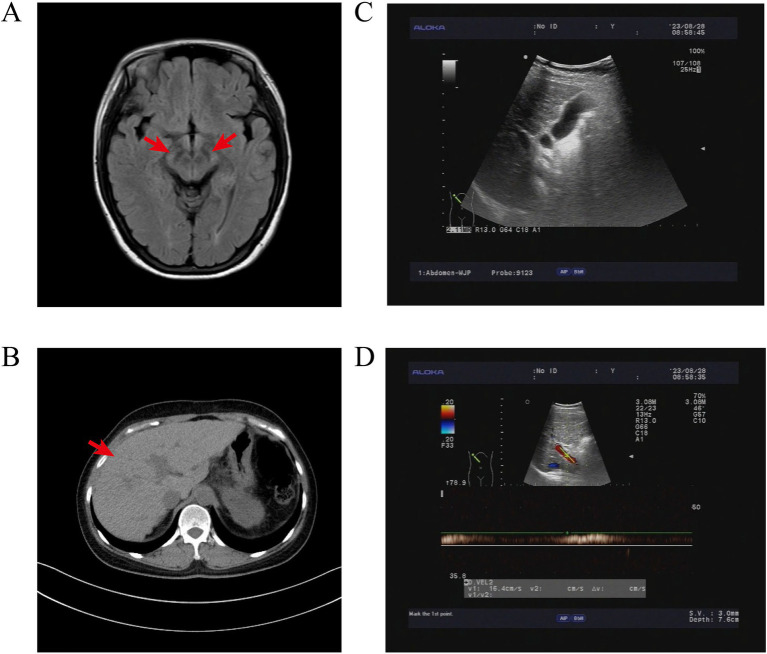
Imaging findings of the patient with WD. (A) Cranial MRI T1WI showed symmetrical abnormal signals in the bilateral cerebral peduncles, consistent with the typical manifestation of central nervous system involvement in WD; (B) chest CT revealed an irregular liver contour, uneven margin, and heterogeneous hepatic parenchymal density; no splenic structure was observed in the splenic region, suggesting post-splenectomy status; (C) liver color Doppler ultrasound demonstrated coarse and enhanced intrahepatic echoes with uneven distribution, suggesting cirrhotic changes in the liver; (D) the portal vein blood flow spectrum was normal (peak velocity [Vmax] = 16.4 cm/s).
